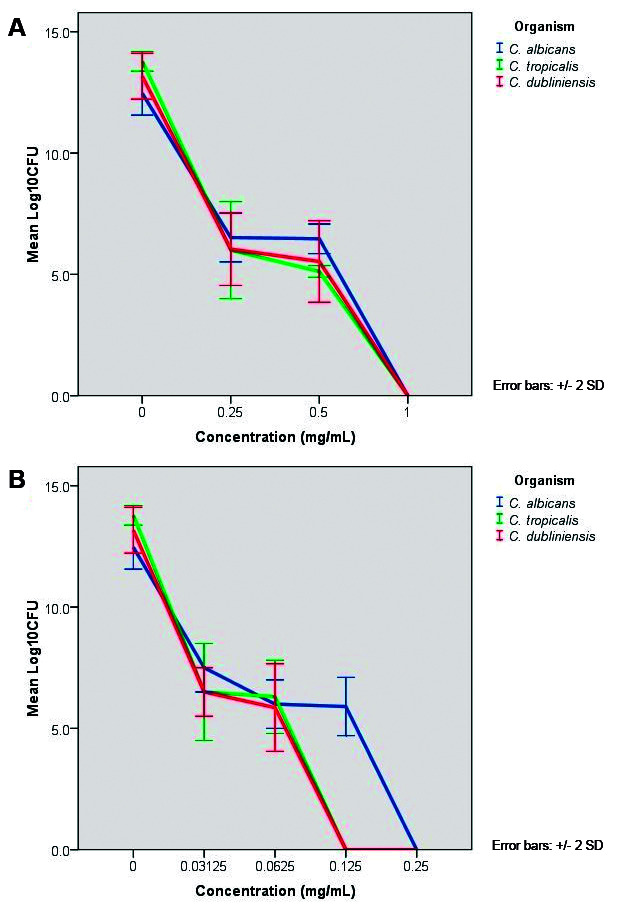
Log colony forming unit (CFU) values of forming *Candida albicans* (CA) (ATCC MYA-2876), *C. tropicalis* (CT) (ATCC 750) and *C. dubliniensis* (CD) (ATCC MYA-646) biofilms in the presence of different concentrations of (A) *Cinnamomum verum* (CV) leaf oil and (B) chlorhexidine digluconate (CHL). All error bars represent the ± 2 standard deviations (SD).
